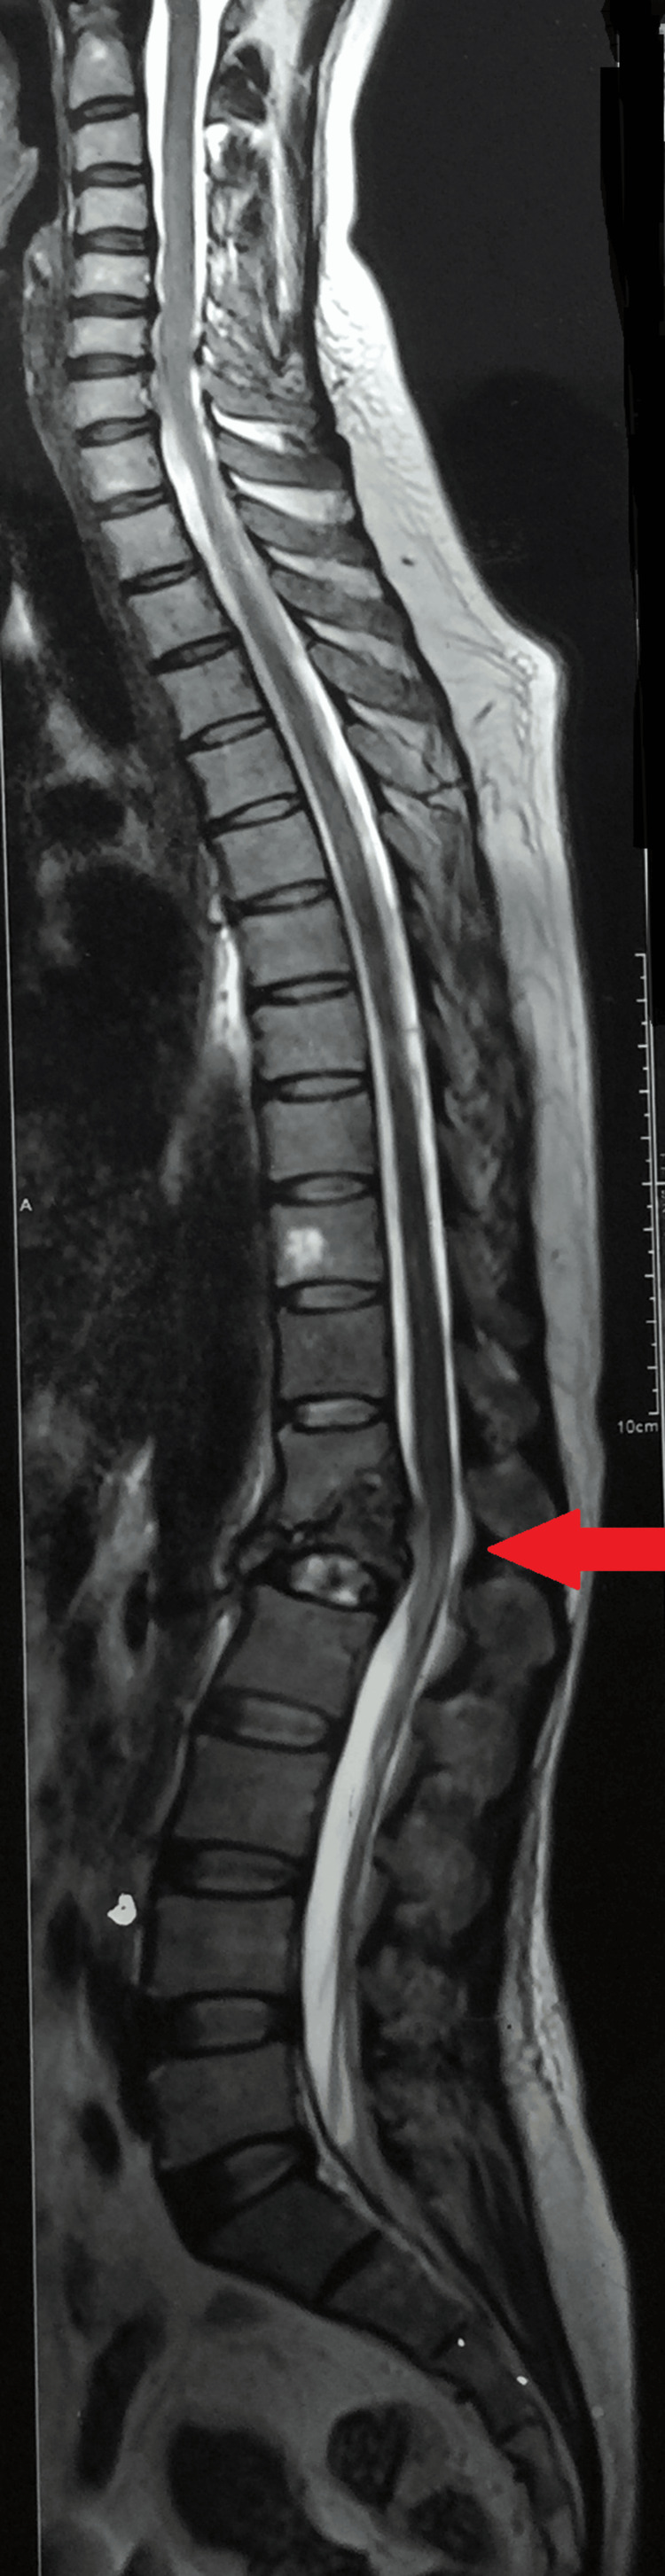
MRI of the dorso lumbar spine showing the collapse of the first lumbar vertebra with cortical destruction and marrow edema with partial collapse of D12 with loss of D12-L1 height with inferior endplate erosion
